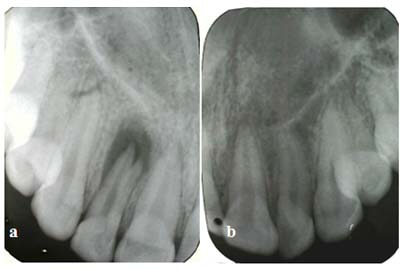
The maxillary right lateral incisor has two roots while the contralateral incisor is single-rooted a: Periapical radiographic image of the maxillary right lateral incisor b: Periapical radiographic image of the maxillary left lateral incisor
